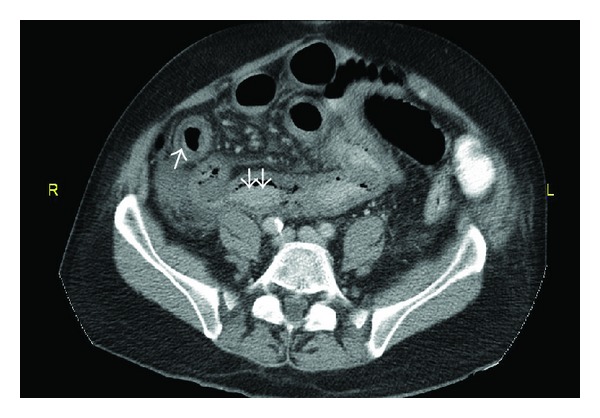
Computed tomography (CT) of the abdomen and pelvis with IV contrast showing marked thickening of the terminal ileum.
